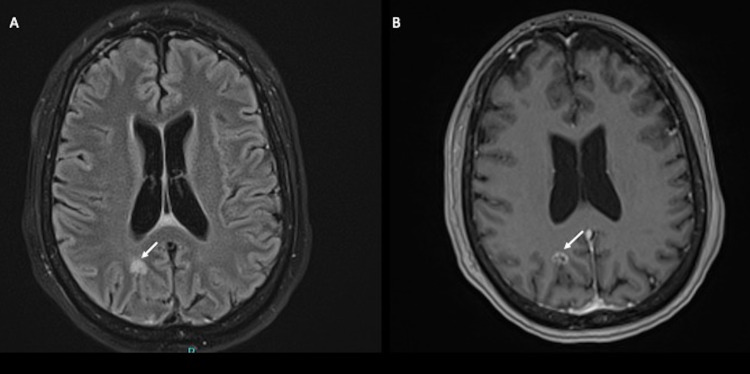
MRI of the brain with and without contrast. Panel A shows the axial T2 FLAIR sequence showing the T2 hyperintense lesion (arrow) in the right occipital lobe. Panel B shows the axial 3D T1 MPRAGE sequence showing the lesion taking up gadolinium contrast in a ring-enhancing pattern (arrow). MRI: magnetic resonance imaging; FLAIR: fluid-attenuated inversion recovery; MPRAGE: magnetization-prepared rapid acquisition gradient-echo
